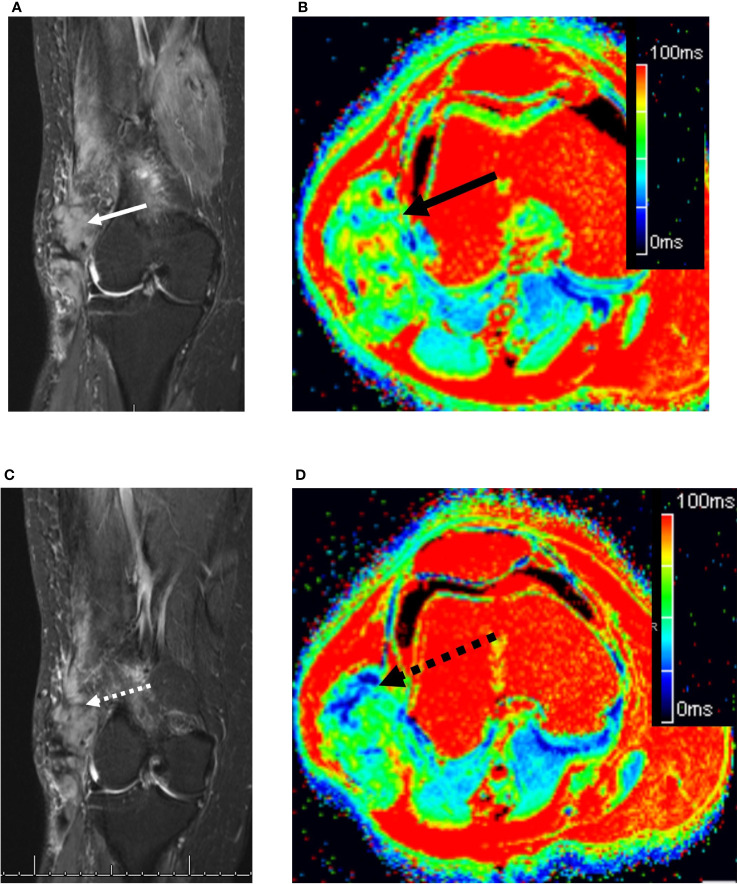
73 year old woman with a right lateral thigh mass. (A) Coronal fat-suppressed T2-weighted MRI demonstrates an ovoid heterogeneously hyperintense desmoid tumor in the lateral distal thigh (solid white arrow). (B) The corresponding axial T2 map demonstrated the mass (solid black arrow) to have a relaxation time of 59.1 ms (readers 1 and 2: 57.5 and 60.7 ms, respectively). (C) Four months later while on sorafenib, the tumor remains unchanged in length, but shows subtle decreased T2 hyperintensity (dashed white arrow). (D) Axial T2 map demonstrates small areas of decreased T2 relaxation times (dashed black arrow), bringing the mean tumor T2 down to 55.5 ms (readers 1 and 2: 56 and 54.9 ms, respectively).
